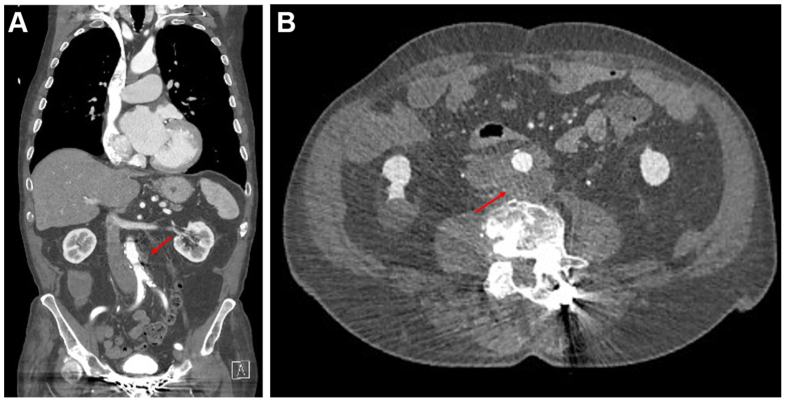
Computed tomography aortogram (CTA) on initial presentation, with *red arrows* pointing to the periaortic mass. (A) Coronal. (B) Axial.
